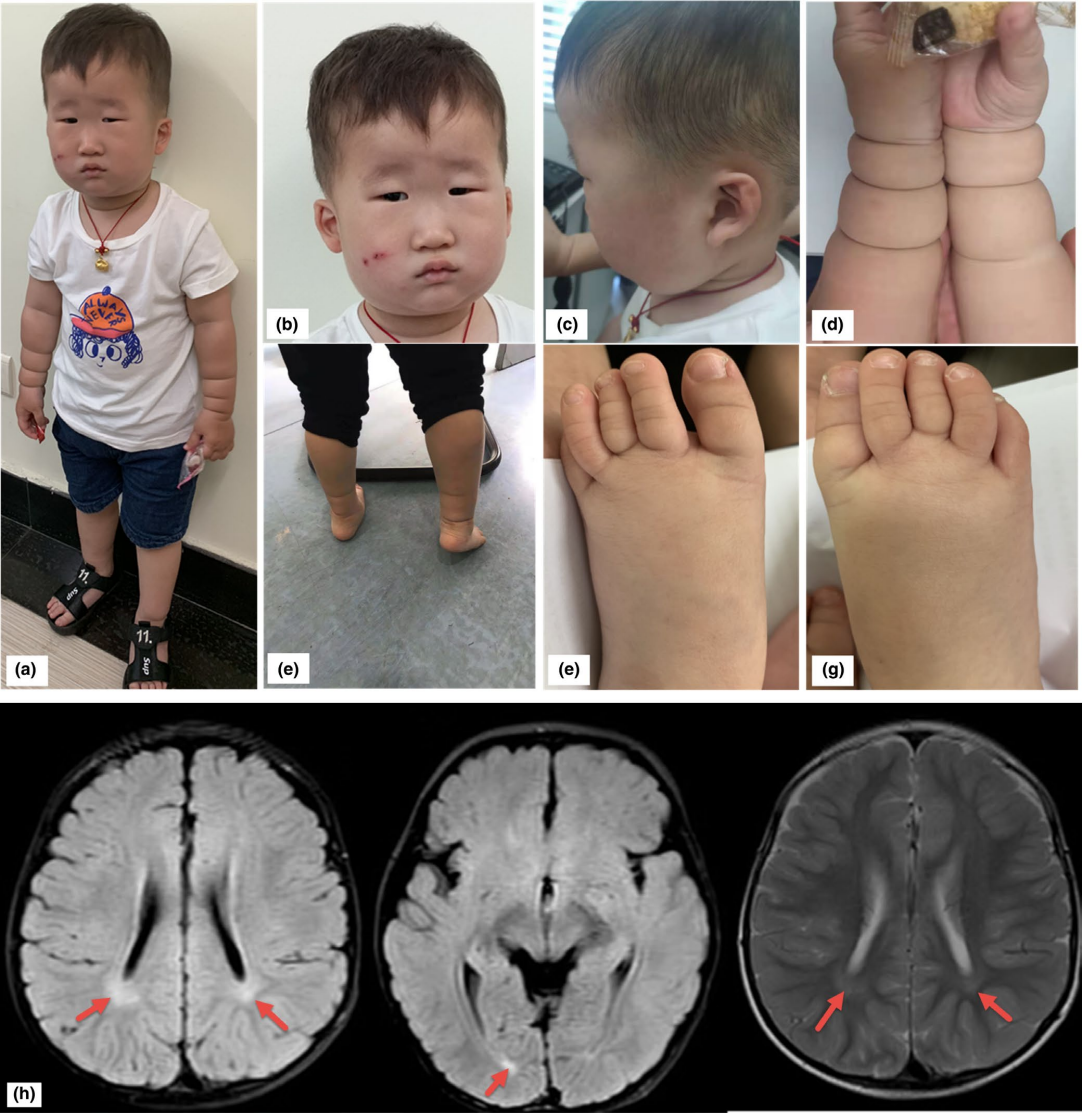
The proband's clinical features at 2 years and 3 months of age. (a) The proband's general appearance. (b) Dysmorphic facial features: macrocephaly, micrognathia, bitemporal narrowing, elongated and flat face, blepharophimosis, broad depressed nasal bridge, epicanthic folds, microphthalmia, microstomia. (c) Low‐set ears with fewer folds. (d and e) Circumferential skin creases on forearms and ankles. (f and g) Apparently partial syndactyly‐like malformation on both feet. X‐ray imaging showing normal. (h) The proband's Magnetic resonance imaging (MRI) demonstrating partial agenesis of white matter of posterior portion
